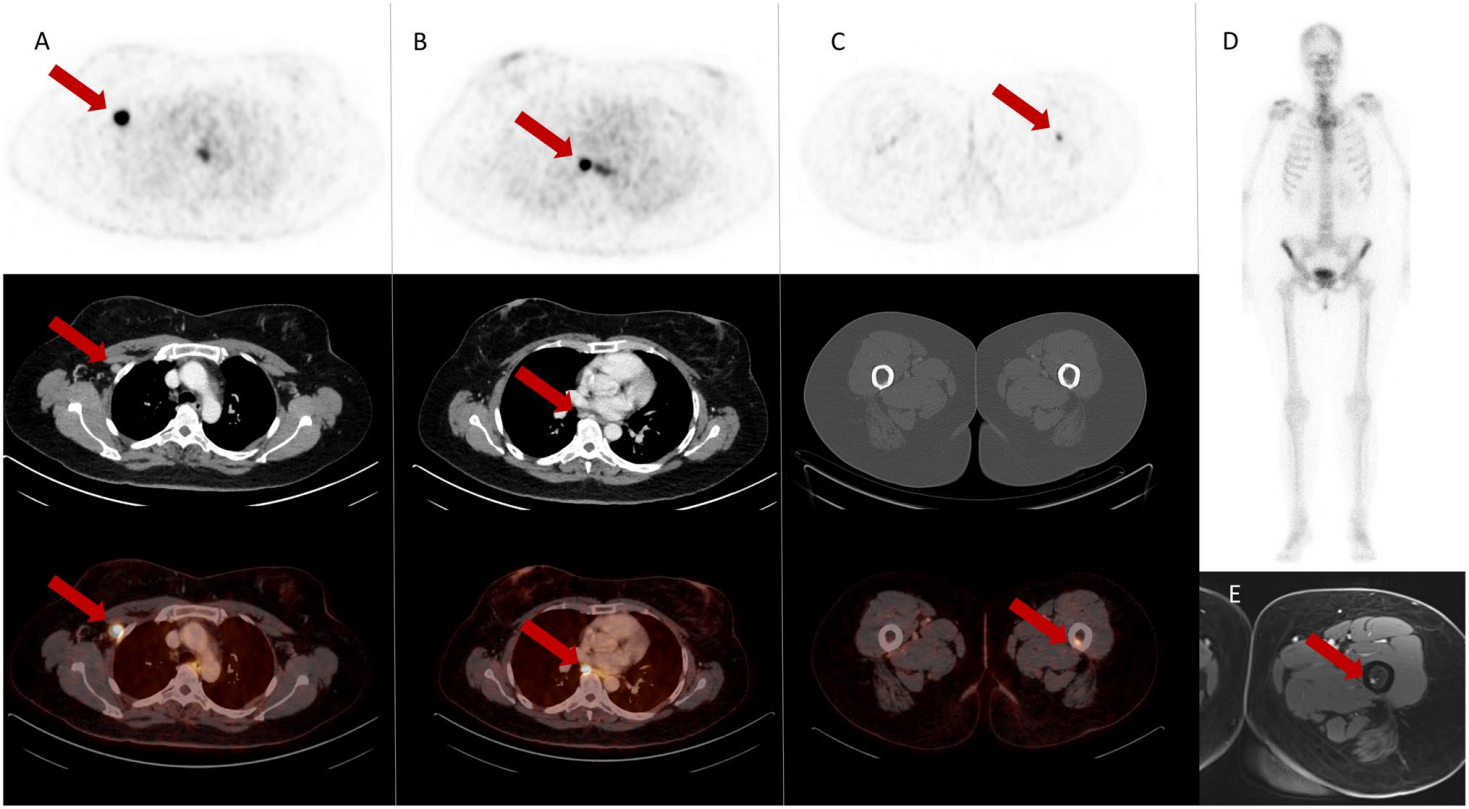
PET/CT imaging with [^68^Ga]Ga-RM2 (A-C; axial slices of PET [top], CT [middle] and fusion images [bottom]). An ipsilateral deep axillary enlarged lymph node with intense tracer uptake was detected, compatible with a local lymph node metastasis A. High tracer uptake was observed in a small subcarinal, distant lymph node metastasis B, confirmed by biopsy. Furthermore, high focal tracer uptake was found in the left proximal femur C. Despite an unsuspicious planar bone scan D, magnetic resonance imaging E was highly suggestive for a bone metastasis
